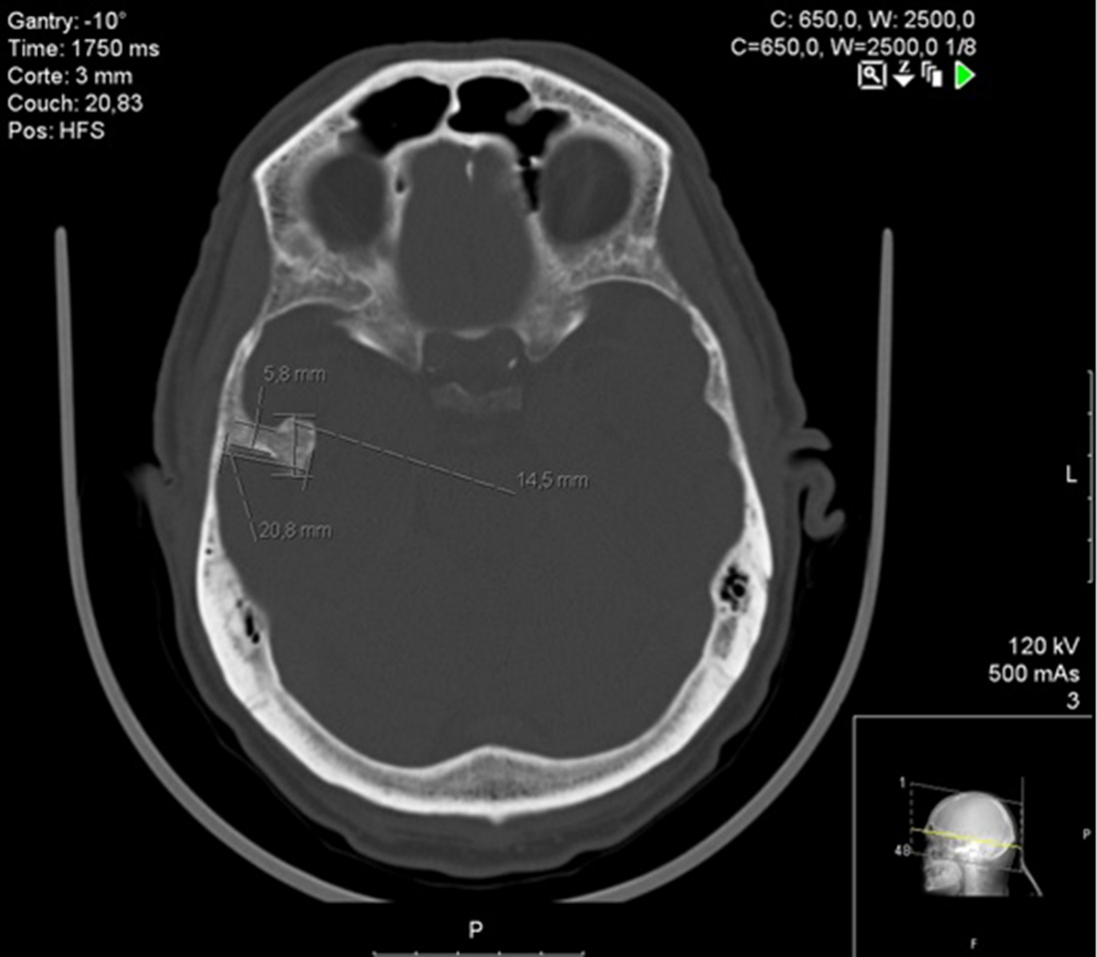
Right temporal enostosic lesion on non-contrast brain CT (bone image).
